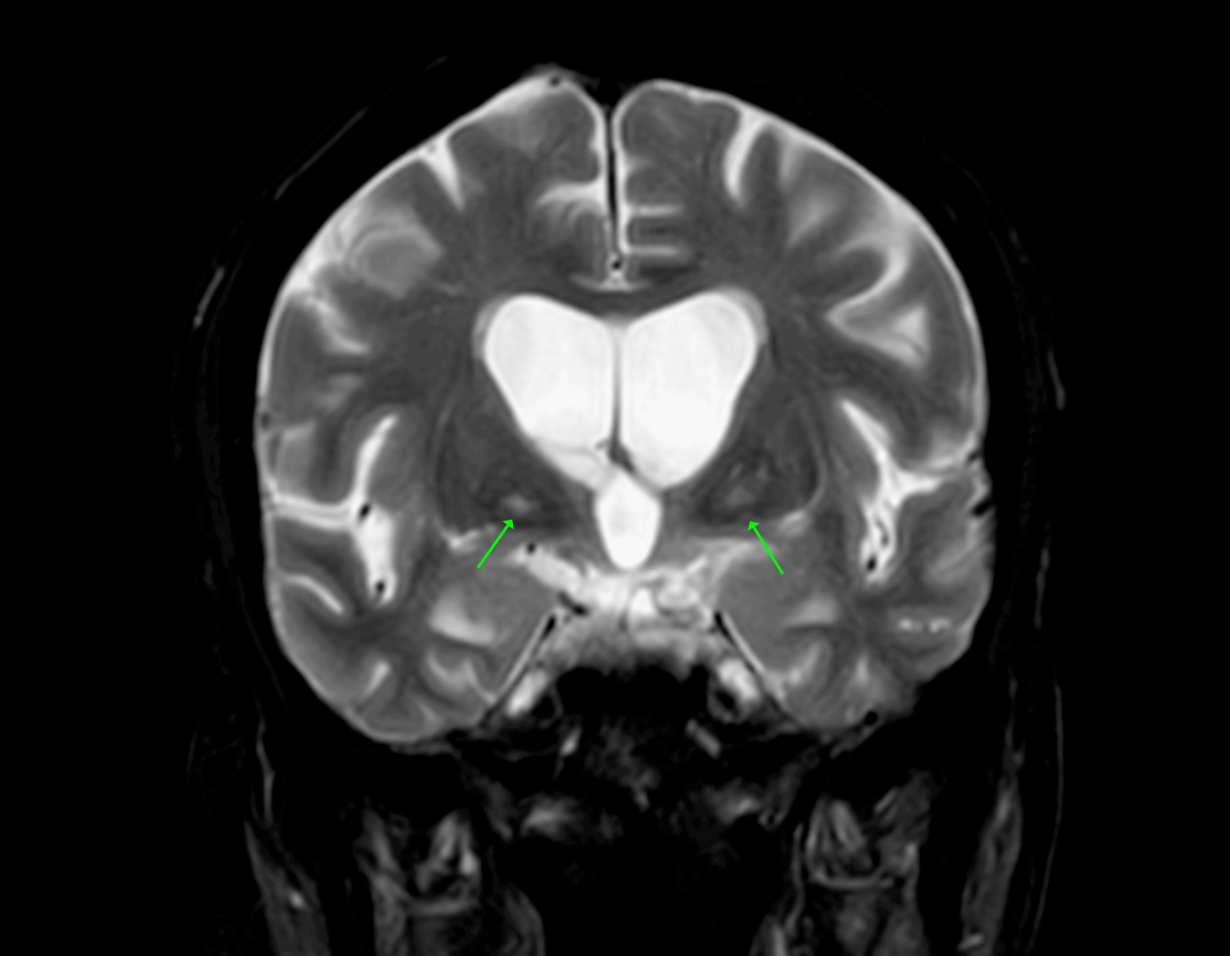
"Eye-of-the-tiger-like" appearance. Coronal MRI T2 Fluid Attenuation Inversion Recovery (FLAIR) image demonstrating "eye-of-the-tiger-like" appearance (arrows).
